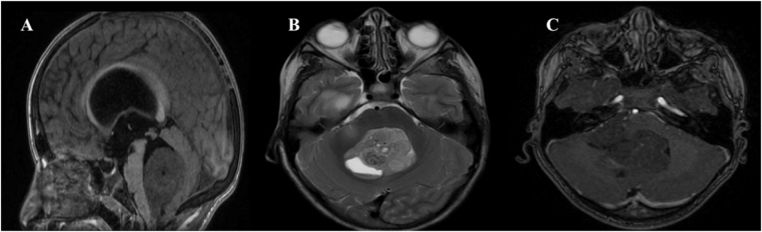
Preoperative MRI of a fourth ventricular tumor. (A) Sagittal T1-weighted image showing a midline posterior fossa mass filling and expanding the fourth ventricle. (B) Axial T2-weighted image demonstrating heterogeneous hyperintensity with a central cystic component. (C) Axial post-contrast T1-weighted image showing heterogeneous enhancement, with secondary compression of the cerebellar vermis and brainstem.
